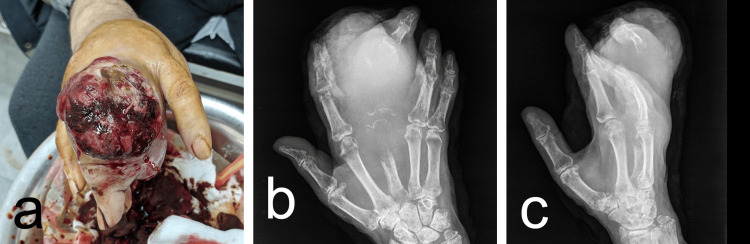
(a) Preoperative image showing the necrotic surface of the tumor with hemorrhagic exudate; (b, c) Preoperative X-rays demonstrating enlargement of the soft tissue mass surrounding the head of the third metacarpal bone and the proximal and middle phalanges of the third finger, extending to involve the adjacent second and fourth fingers, and accompanied by bone lysis.
